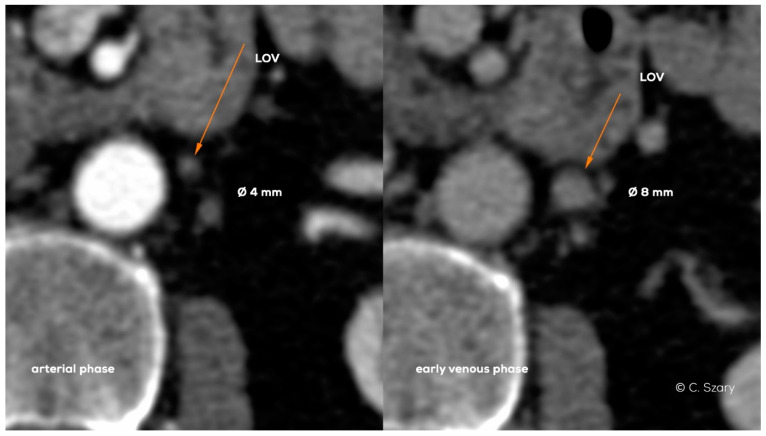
Axial CT images in arterial and early venous phase show significant difference in left ovarian vein (LOV) diameters.
